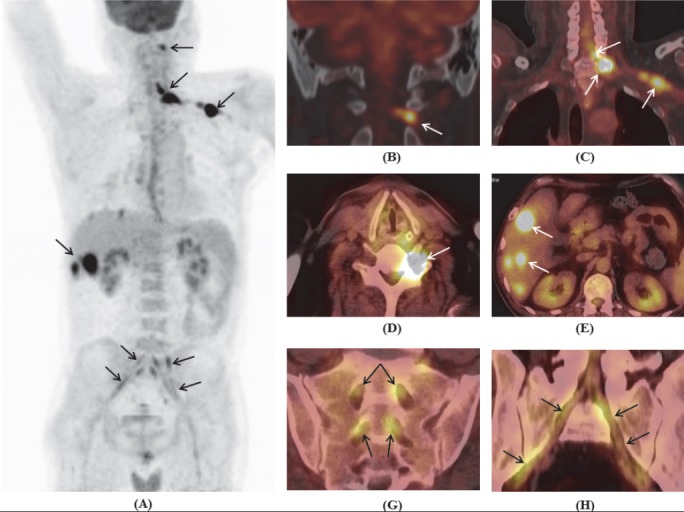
Maximum intensity projection of whole body (A) and PET/CT images (B-H) showed increased FDG uptake in the left C2 nerve root (B); C5-C6 spinal cord, C7 nerve root, brachial plexus (C,D); multiple focal lesions in liver (E); and bilateral lumbosacral plexuses and along the sciatic nerves (G,H)
